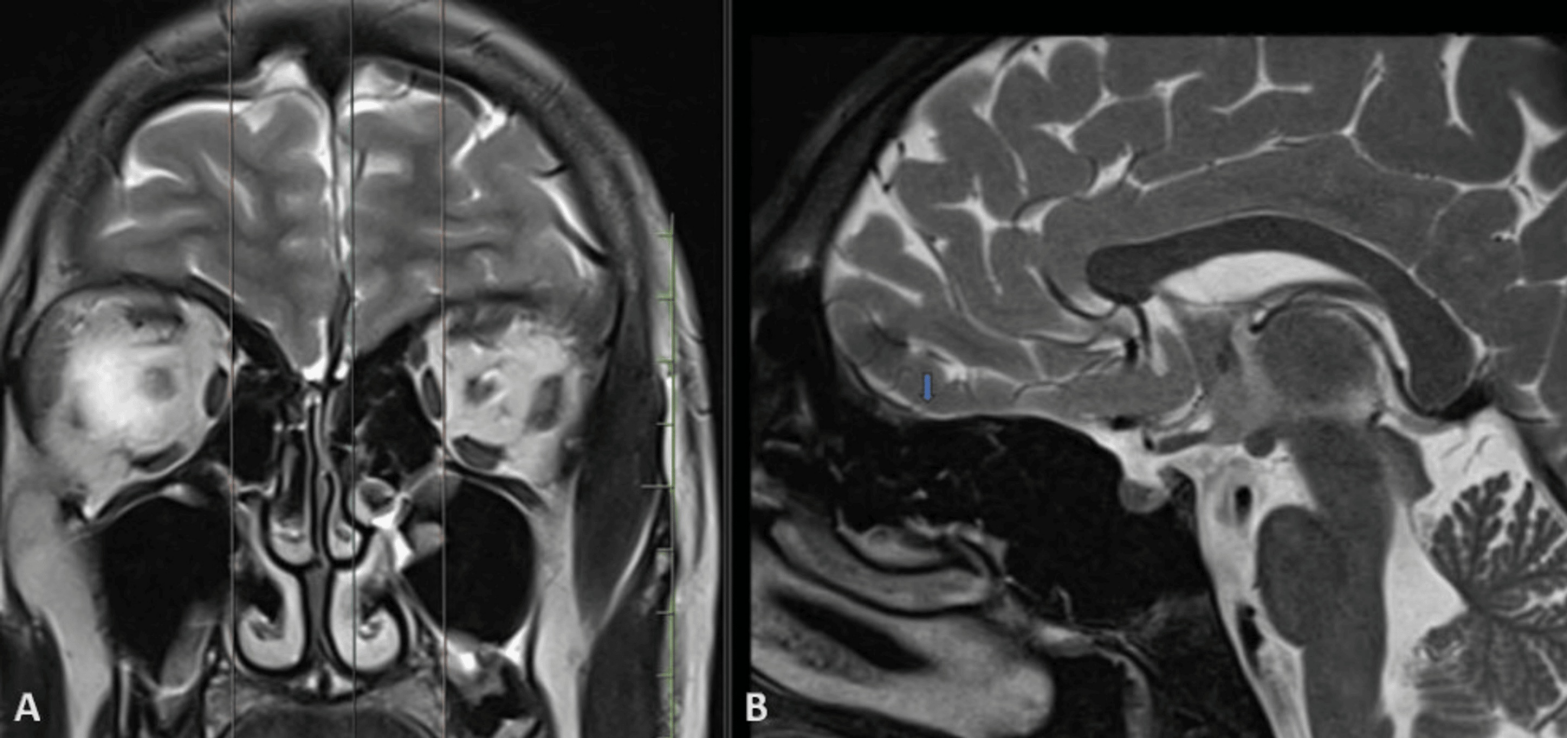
Coronal T2-weighted MRI in coronal (A) section with sagittal section (B) at corresponding level show absent olfactory bulb in left olfactory groove with hypoplastic olfactory sulcus (blue arrow).
